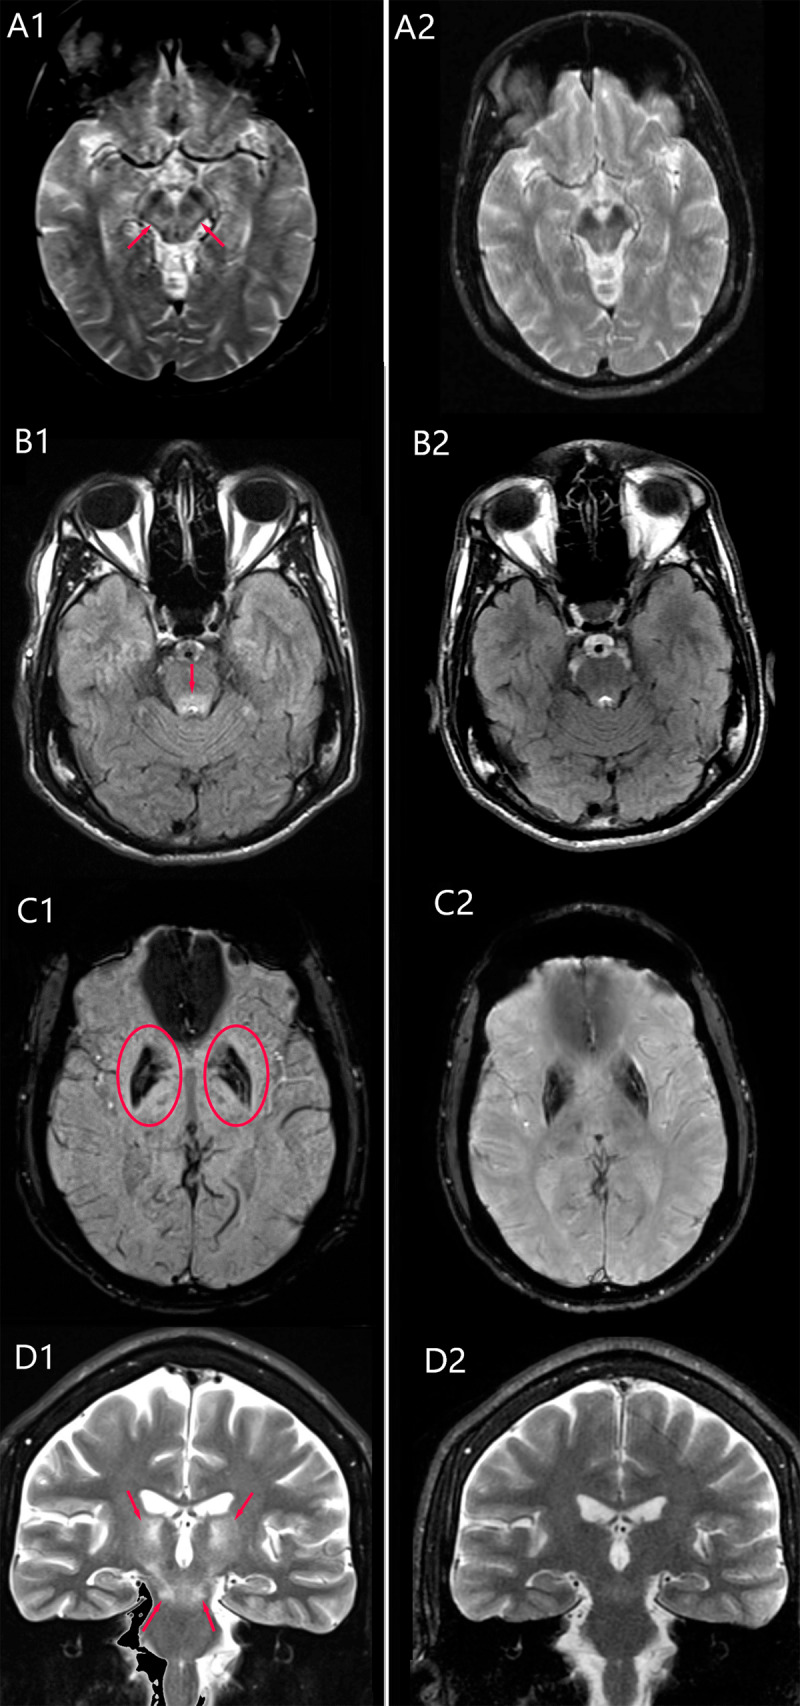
Left: Wilson’s disease brain MRI before treatment. MRI shows T2-hyperintensity in the midbrain tegmentum (red arrows in A1), pontine tectum (red arrow in B1), and dentato-rubro-thalamic tract (red arrows in D1). SWI reveals hypointensity and atrophy in the lentiform and caudate nuclei (red circles in C1). Right: Brain MRI post 3-year penicillamine (A2–D2). Marked improvement in T2 hyperintense lesions.
